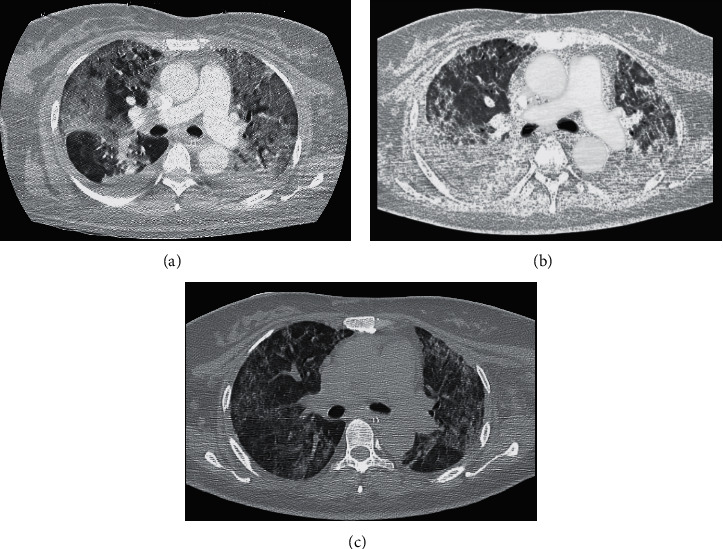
Axial chest computed tomography scan. (a) Before the first extracorporeal membrane oxygenation support. Diffuse bilateral ground glass opacity pattern and right pleural effusion are shown. (b) During the first extracorporeal membrane oxygenation support. Thickening of interstitial septa and diffuse crazy paving are shown. (c) After the first decannulation of extracorporeal membrane oxygenation. Lung aeration improved bilaterally with partial resolution of ground glass opacities.
